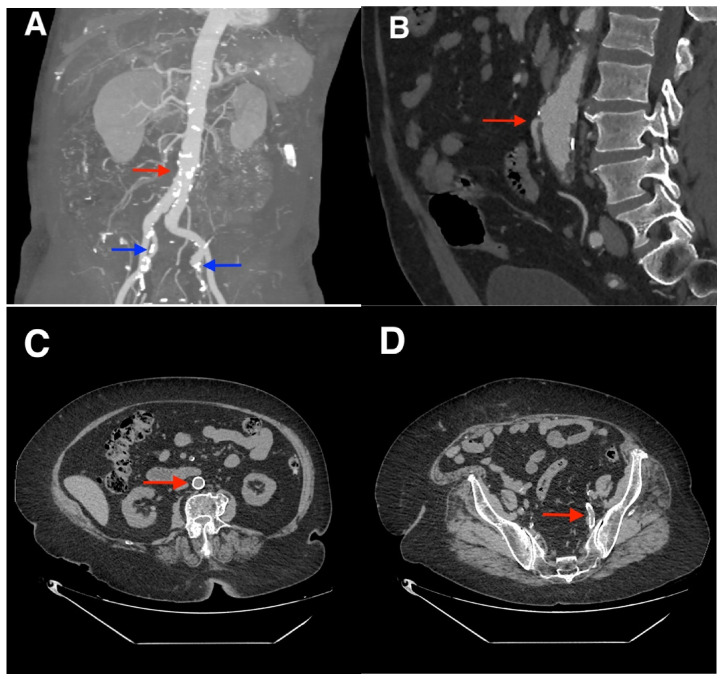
Examples of CT-derived vascular disease markers. (A) 3D maximum intensity projection reconstruction of the abdominal vessels showing significant calcific burden predominantly concentrated in the infrarenal abdominal aorta (red arrow) and the internal iliac arteries (blue arrows). (B) CT angiography showing stenosis of the inferior mesenteric artery (red arrow). (C) Non-contrast CT image at the L3 vertebral level showing calcifications covering the entire circumference of the inferior abdominal aorta (red arrow). (D) Same examination: the red arrow points to calcifications involving the internal iliac artery.
